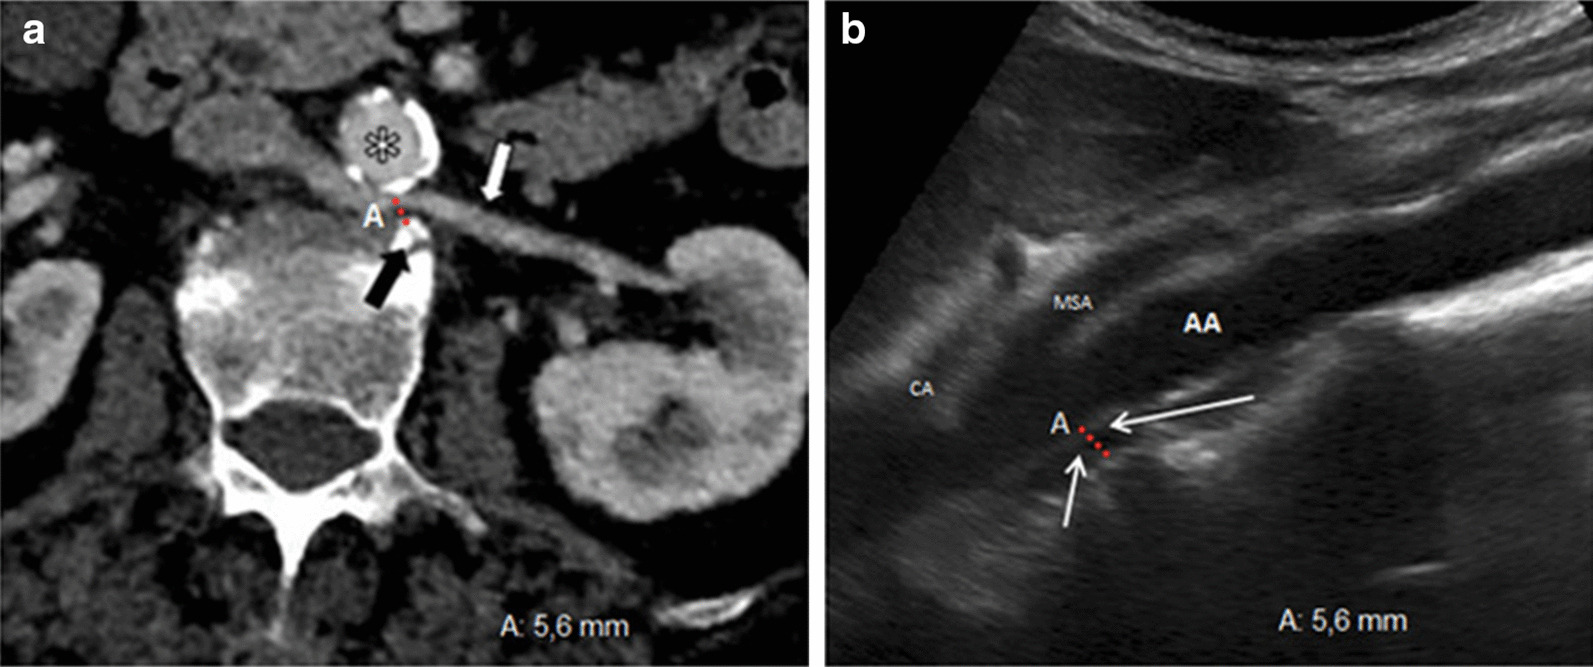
Aorto-vertebral space. a Measurement of the aorto-vertebral distance (A) with multi-detector computed tomography. Retro-aortic left renal vein (white arrow). Abdominal aorta (*). Osteophyte (black arrow) of the third lumbar vertebral body. b Measurement of the aorto-vertebral distance (A) with B-mode ultrasound. Left renal vein (short arrow). Aorto-vertebral space (long arrow). AA = abdominal aorta; MSA = mesenteric superior artery; CA = celiac artery
